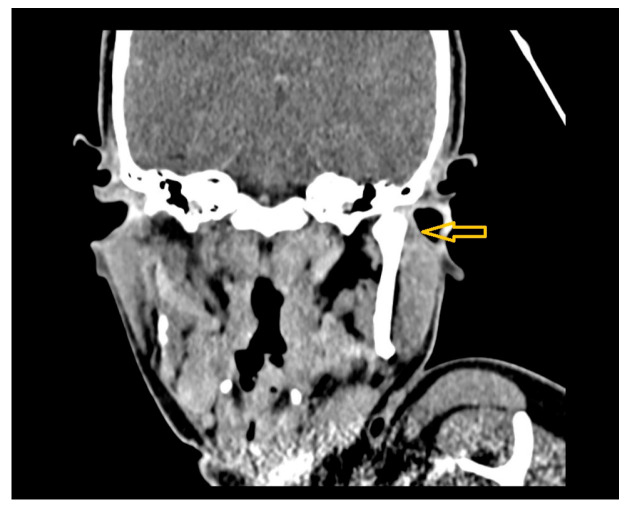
Coronal CT image showing subluxation of the left temporomandibular joint (TMJ), accompanied by surrounding soft tissue swelling extending toward the external auditory canal (yellow arrow).
